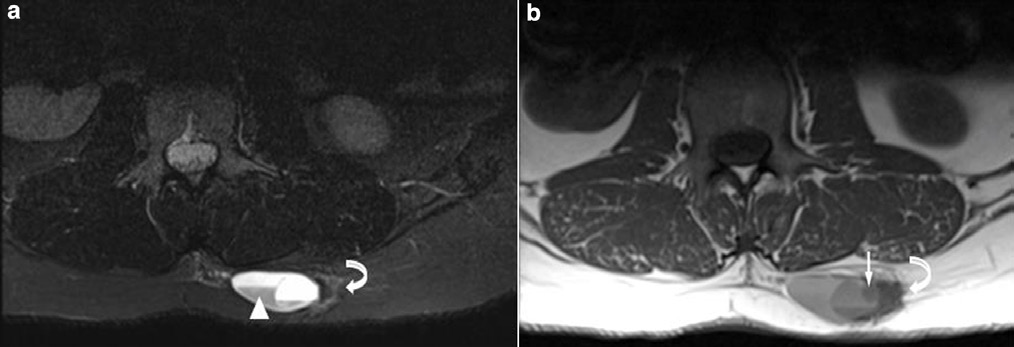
(a) Axial T2 STIR & (b) Axial T1 MR images show a well-defined subcutaneous lesion containing fluid–fluid levels (white arrowhead) and an internal solid nodule (white arrow) with ill-defined low signal change in the adjacent subcutaneous fat on the lateral margin (curved arrow). STIR, short tau inversion recovery.
